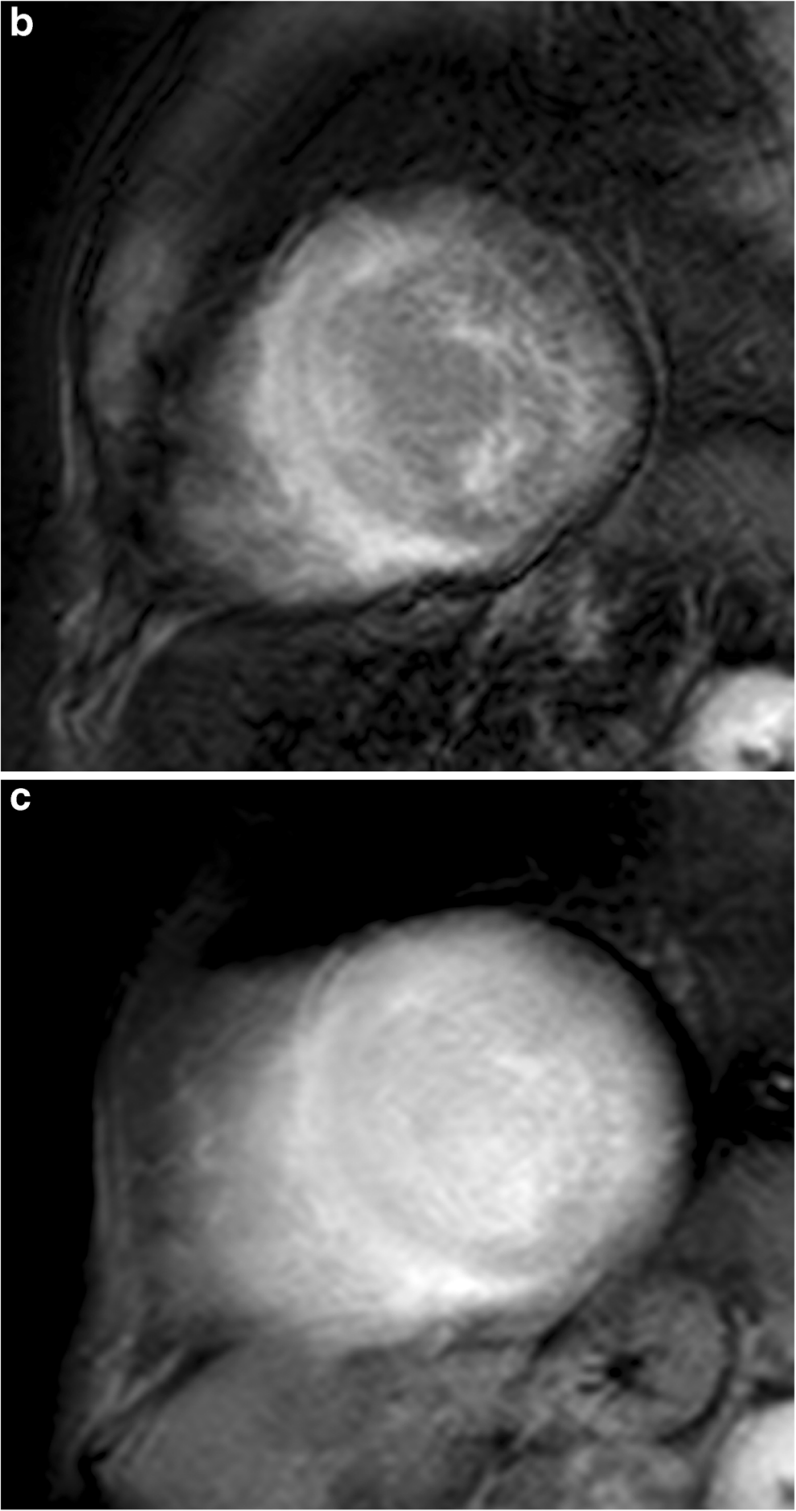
A 75-year-old man with cardiac amyloidosis. a Sequential inversion recovery T1-weighted gradient echo images with a variable inversion time trying to null normal myocardium show the difficult to determine the optimal inversion time to null myocardium. The blood pool and the myocardial nulling occurs earlier at 225 ms than the splenic nulling (275 ms). b Short-axis T1-weighted inversion recovery gradient echo images obtained at (b) 4 minutes and (c) 8 minutes after the injection of contrast agent. The myocardium displays predominantly subendocardial LGE at 4 minutes, but it is diffusely enhanced at 8 minutes
